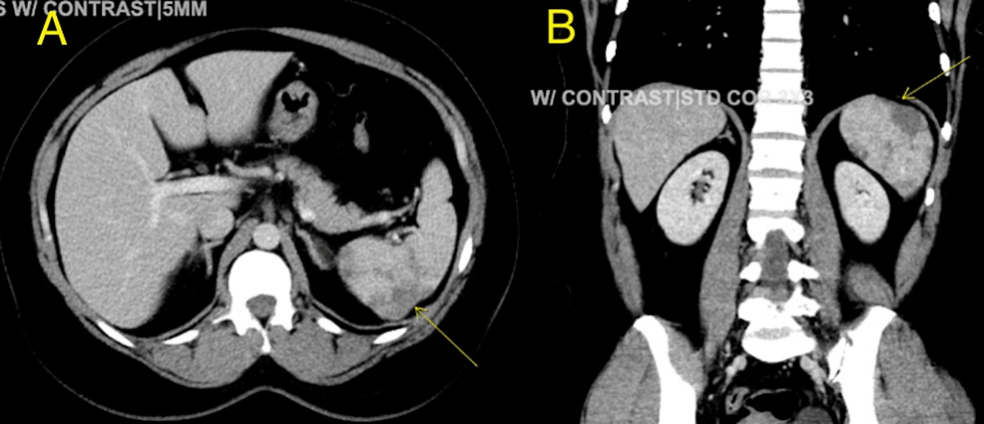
CT abdomen pelvis with contrast axial (A) and coronal (B) view showing splenic infarcts (yellow arrow)
